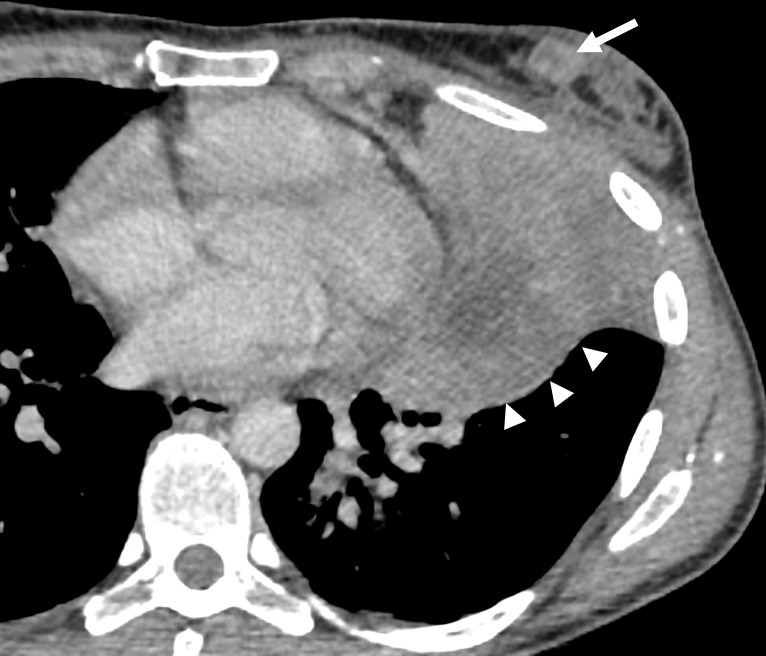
Axial CT-scan showing the breast nodule (arrow) and the lung lesion (arrowheads).
